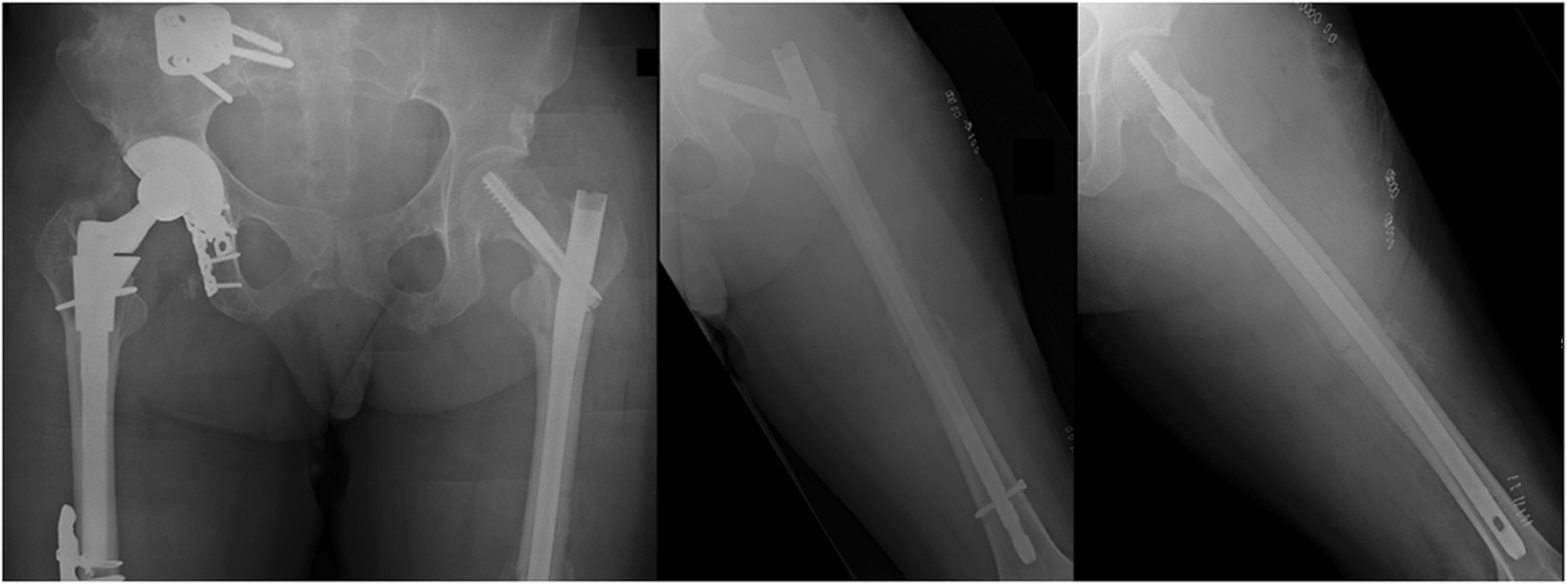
Plain radiographs after the initial surgical revision with reduction and placement of a cephalomedullary nail for fixation of the femoral neck fracture and ipsilateral femur shaft fracture. The fracture appears to be slightly malreduced in varus of the hip.
